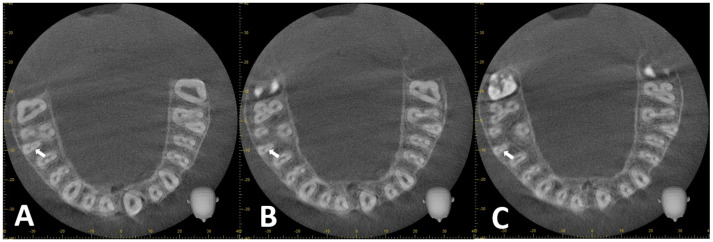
CBCT reconstructions of MB root (arrow) in maxillary first molar tooth. (A–C) Coronal to apical axial sections exhibiting Vertucci type IV RCC.
